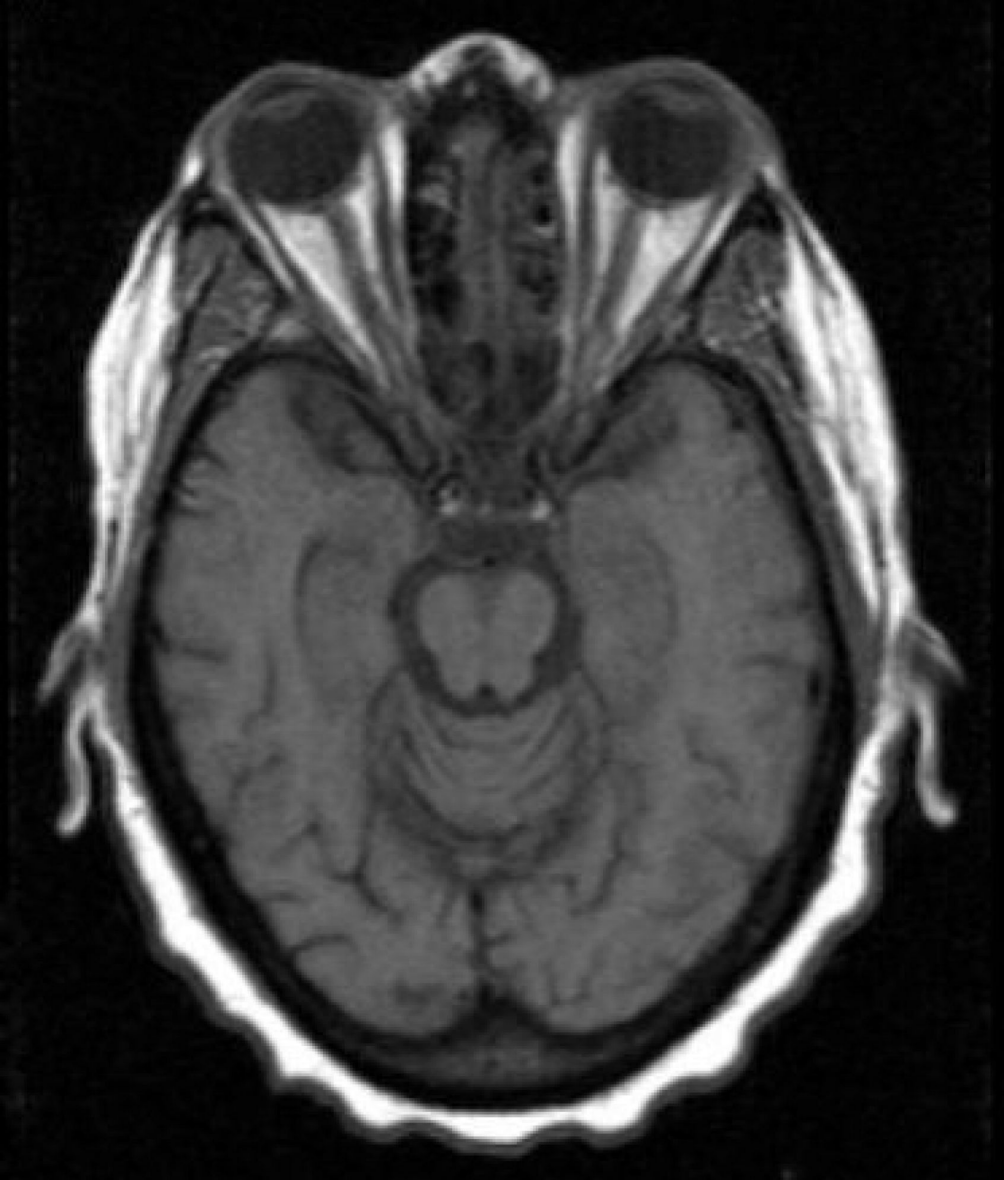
The axial T1-weighted MRI after intravenous injection of gadolinium shows marked signal enhancement of both optic nerves, indicating disruption of the blood-nerve barrier and optic neuritis
